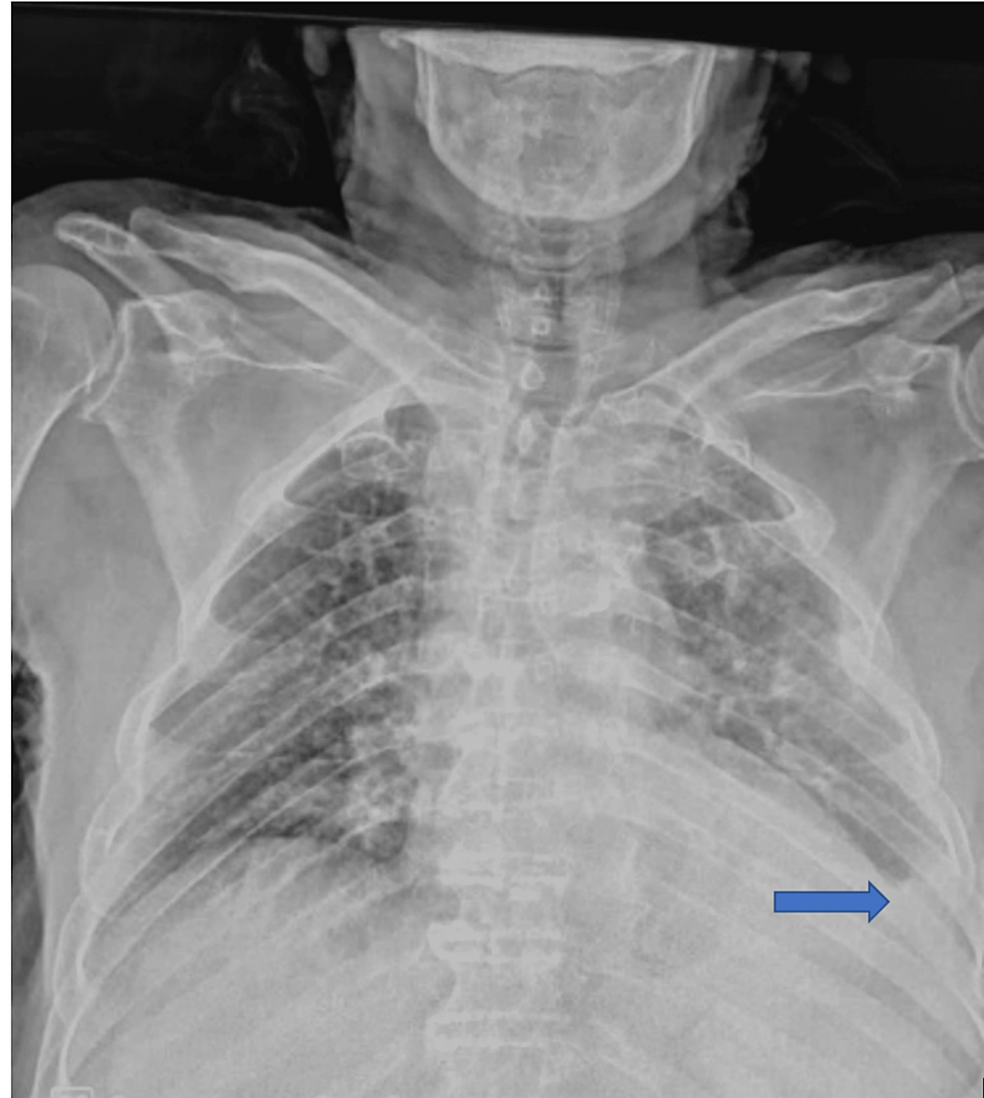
Chest X-ray showing a low-volume left-sided pleural effusion (blue arrow).
